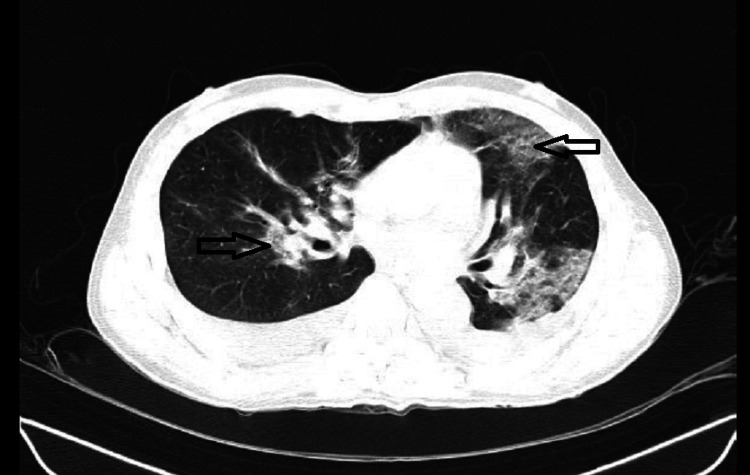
HRCT thorax revealing multifocal areas of ground glass appearance on both sides and pulmonary contusion on the left side HRCT: high-resolution computed tomography
